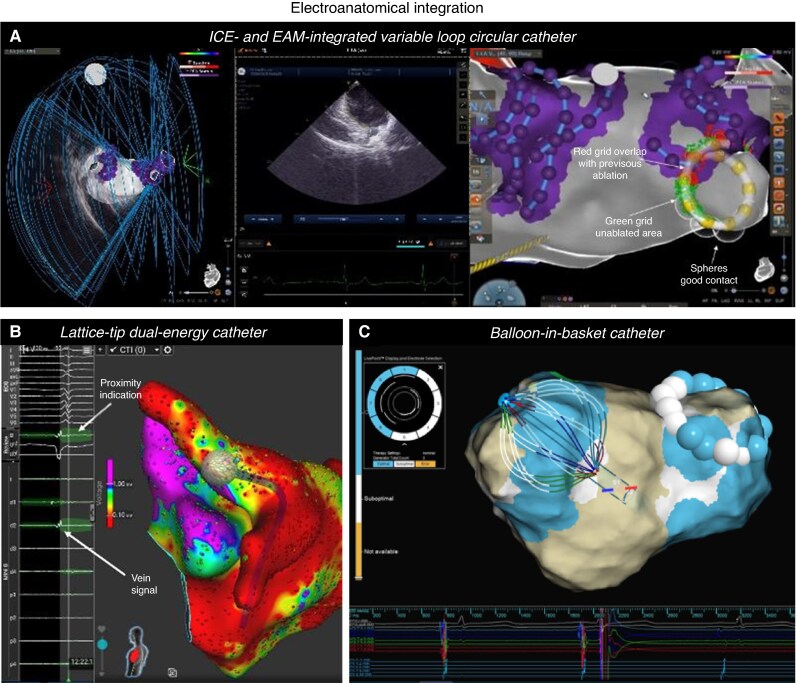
Examples of electroanatomical integration of different pulsed field ablation systems. (*A*) Left and central images: 3D anatomical volume reconstruction using sequential intracardiac echocardiography projections. The image on left side shows ablated zones using the variable-loop circular catheter in purple. Right image: spheres give accurate indication of electrode contact (tissue proximity indicator): sphere dimension is proportional to amount of contact. Small dots predict where energy will be delivered; red indicates lesions overlapping, green unablated tissue. (*B*) Pulmonary vein signals were detected in a redo case using a large footprint lattice-tip catheter. The lattice tip enables high-resolution recording of near-field signals while effectively filtering out noise and far-field activity. Moreover, ablation can be performed directly with the same catheter. The figure also shows the proximity indicator for the single electrode, which helps ensure both accurate signal acquisition and effective lesion formation during ablation. (*C*) Balloon in basket PFA system with electroanatomical mapping integration (LivePoint™ Display Algorithm), which allows for the evaluation of impedance-based lesion-effect parameters through AutoMark lesions (that follow the Livepoint software colour legend) and eField integration. Energy field (eField) displays projection of the electric field of therapy delivery on the map. ICE, intracardiac echocardiography; PFA, pulsed field ablation.
